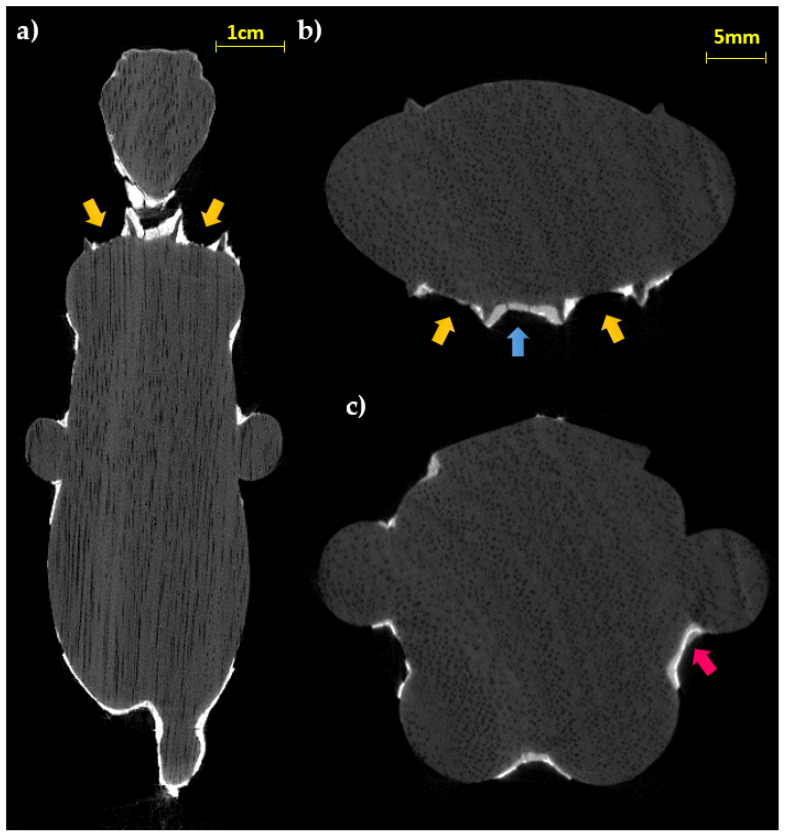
CT longitudinal (a) and horizontal (b,c) slices (yellow arrows: wing grooves; blue arrow: fractures inside the preparation layer; pink arrow: material layer above the gold leaf).
